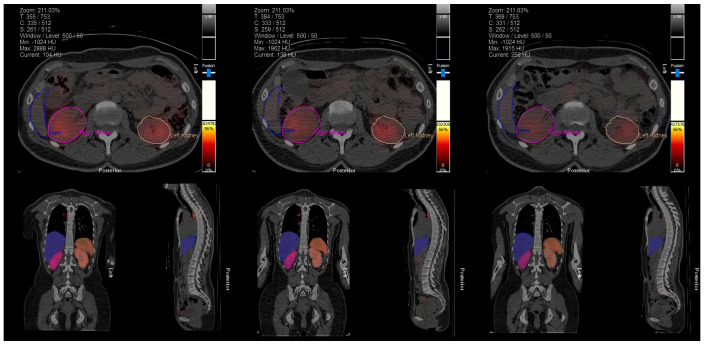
Example of image-based dosimetry in a patient undergoing ^177^Lu-DOTATATE PRRT. Quantitative SPECT/CT acquisitions were performed at 24, 96 and 192 h after radiopharmaceutical administration. The liver was segmented in blue, the left kidney in yellow, the right kidney in pink and the spleen in orange.
